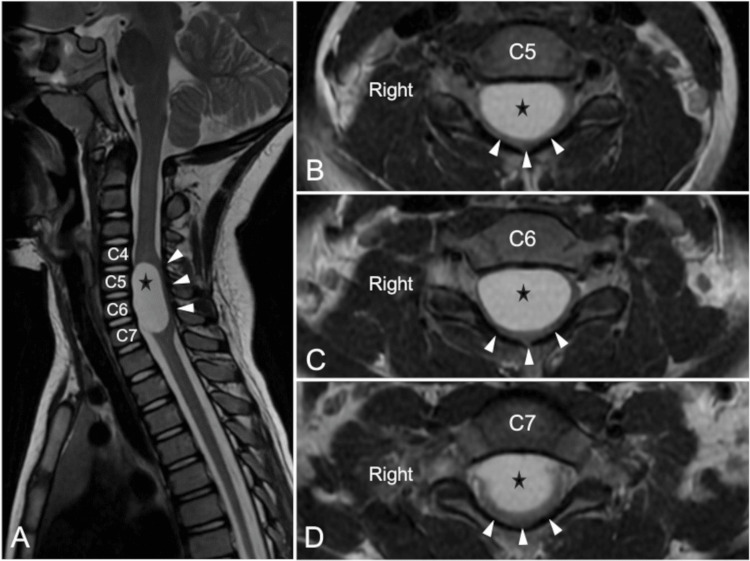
Preoperative cervical magnetic resonance images An intradural extramedullary cystic lesion (black star) at C4-C7 was disclosed. The lesion (black star) caused significant anterior displacement and compression of the spinal cord (white arrowheads), as evidenced by signal changes on T2-weighted imaging (A: a sagittal T2-weighted image; B-D: axial T2-weighted images).
